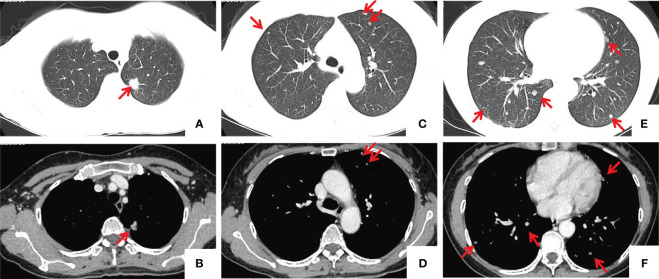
Chest CT images. (A, B) A nodule located in the apicoposterior segment of the left upper lobe, approximately 1.3x1.4 cm in size, exhibits spiculation and lobulation, presenting moderate heterogeneous enhancement and causing adjacent pleural traction. (C–F) Multiple nodules of varying sizes are present in the upper and lower lobes of both lungs (indicated by red arrows), with the larger ones measuring approximately 1.0 cm in diameter, showing mild enhancement. the mediastinal lymph nodes were normal.
